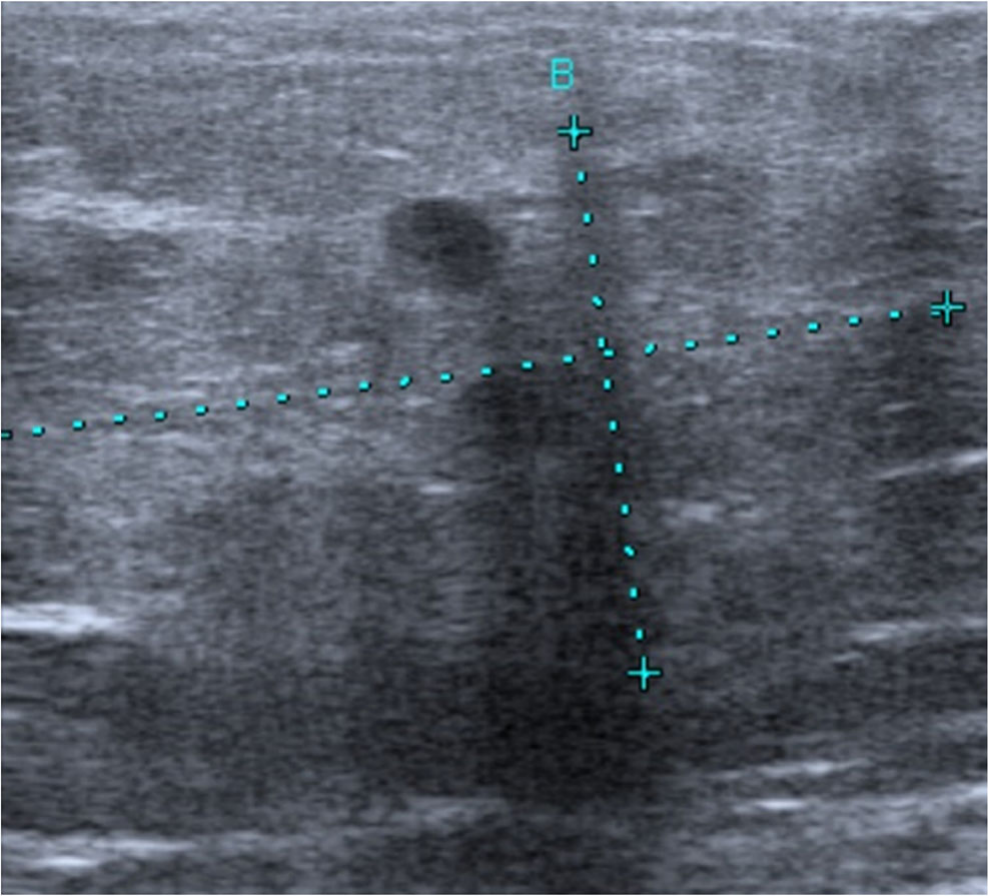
Haematoma. Patient followed for right breast cancer treated in 1998, referred for opinion concerning a mammographic opacity of the right breast not present on the previous mammogram. Ultrasound revealed a mixed hyperechoic and hypoechoic, partially attenuating zone. On clinical interview, the patient reported a motor vehicle accident with ecchymosis of the breast in contact with the safety belt. Ultrasound-guided microbiopsy demonstrated fat necrosis and no suspicious image. Regression of the image on regular follow-up examinations
